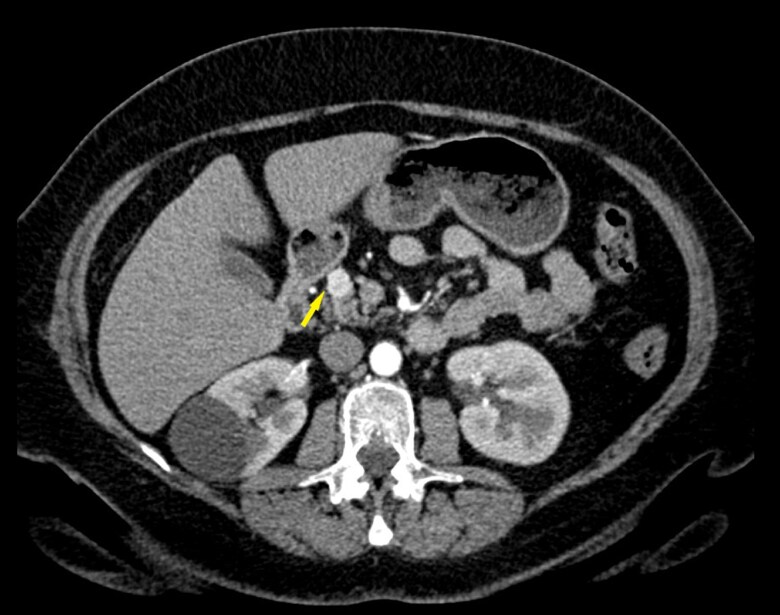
Contrast-enhanced computed tomography of the abdomen demonstrating a 1.4-cm mass in the pancreatic neck (arrow).
